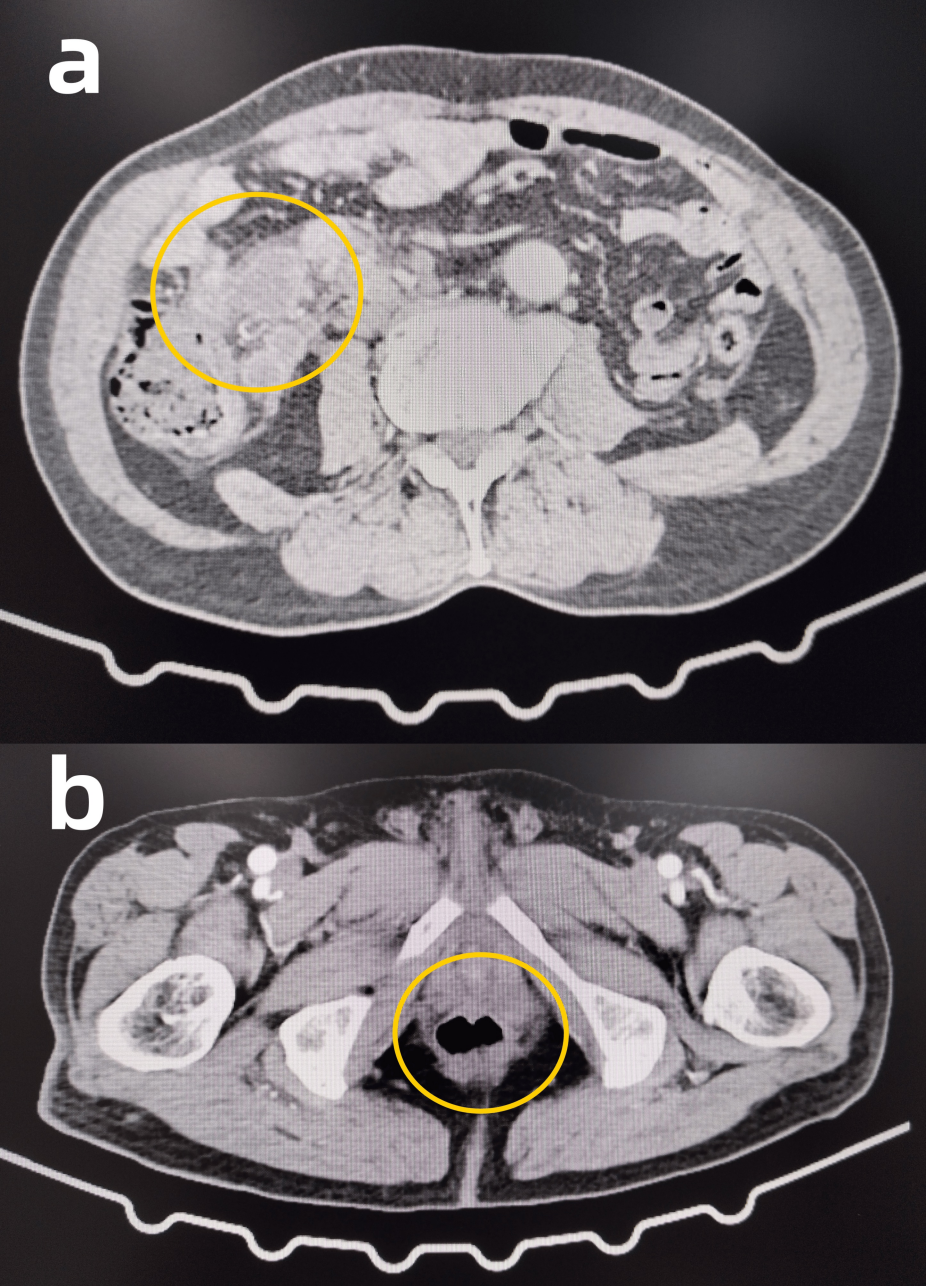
Axial CT scan of the abdomen (a)The yellow circle indicates mass in the right colon and mesenteric region. (b)The yellow circle indicates a rectum mass. CT: computed tomography
